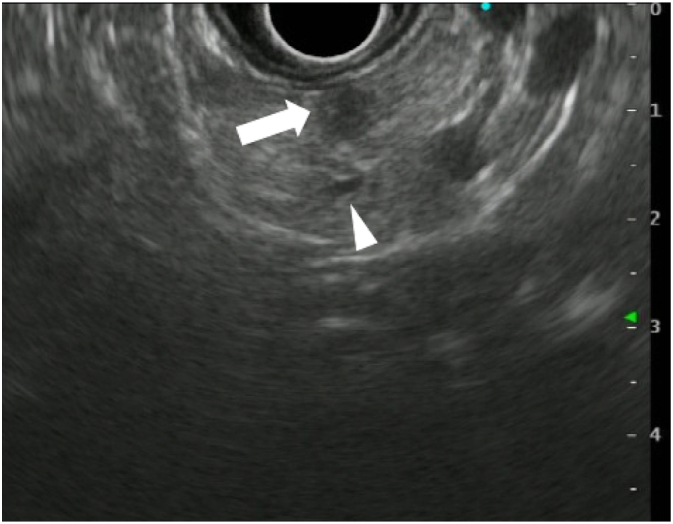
Preoperative endoscopic ultrasonography revealed that the distance between the tumor (arrow) and the main pancreatic duct (arrowhead) was approximately 3 mm.
